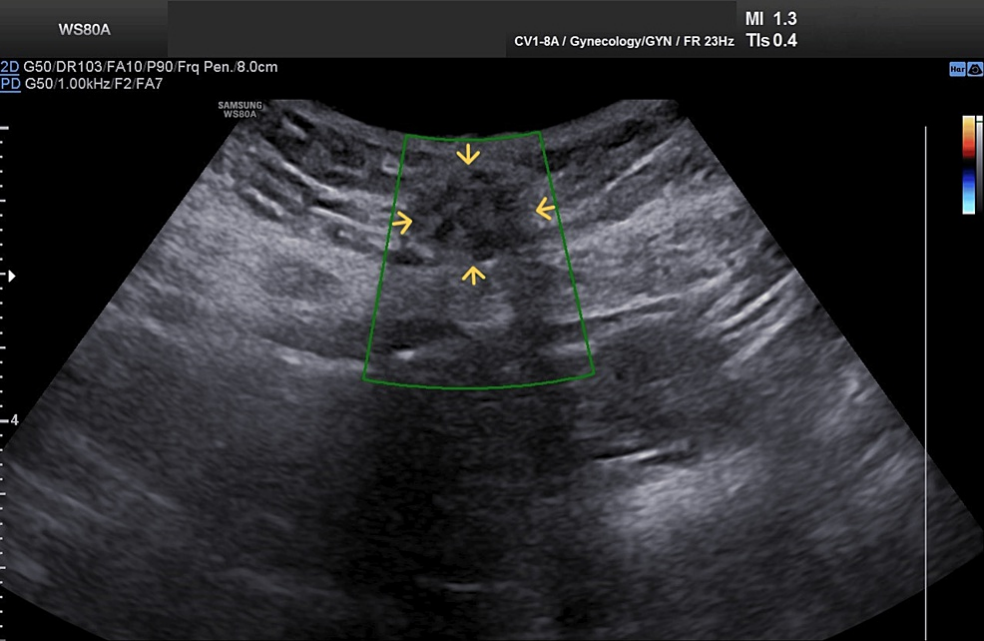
Ultrasound imaging of abdominal wall endometriosis A mixed echogenicity lesion (yellow arrows) is depicted within the subcutaneous tissue, with no evidence of vascularity on Doppler examination
